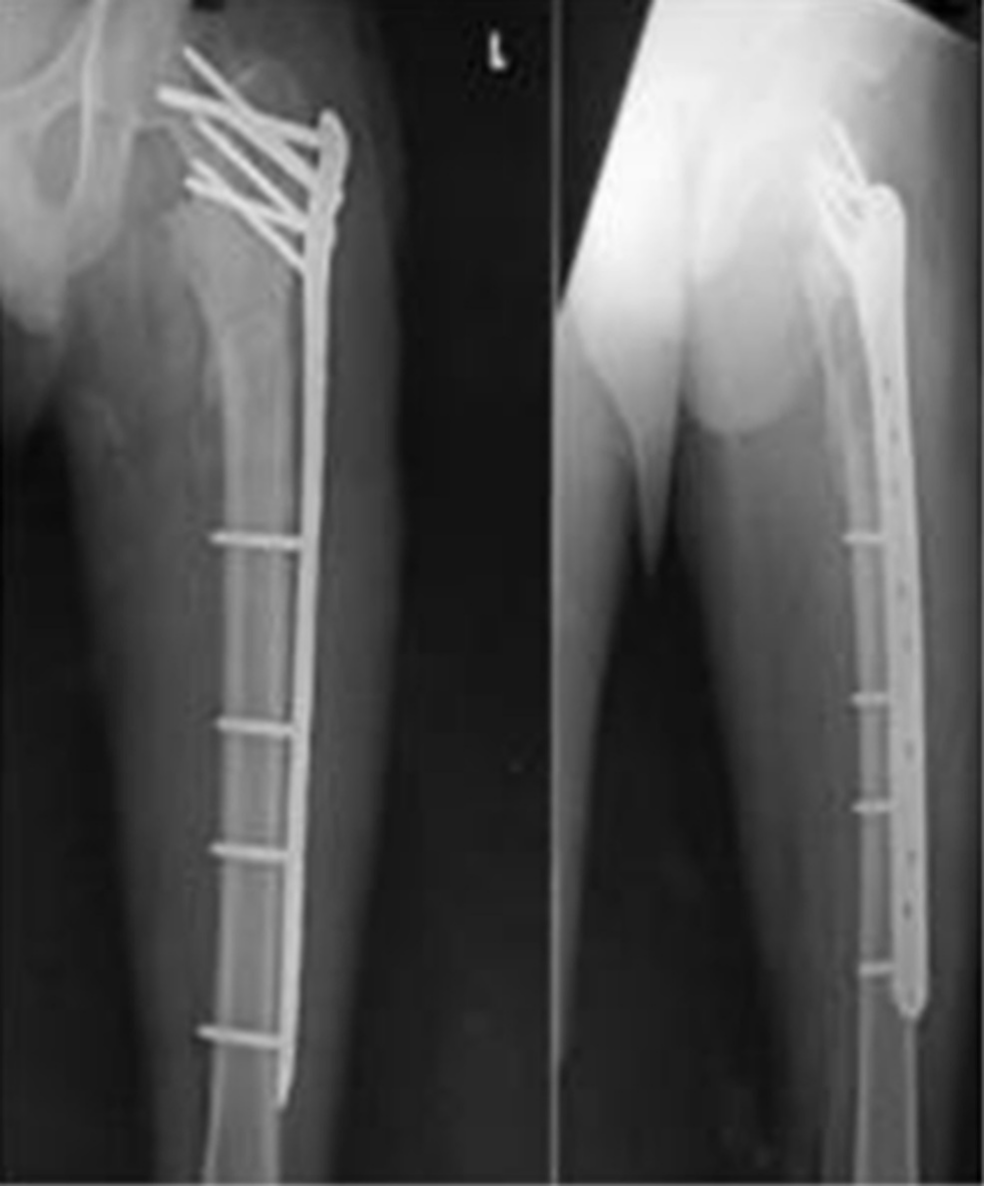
Antero-posterior and lateral radiographs at three months of follow-up
